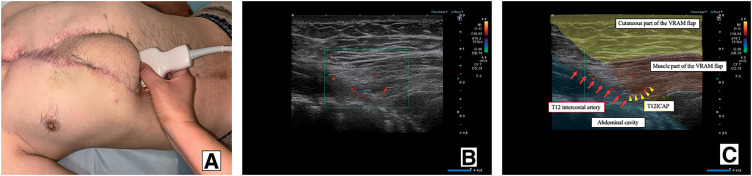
Fig. 4 (A) Postoperative color Doppler ultrasonography was performed to evaluate the flow of T12ICAP. (B, C) Postoperative assessment confirmed reliable perfusion through the T12ICAP to the transferred VRAM flap. T12ICAP: 12th intercostal artery perforator; VRAM: vertical rectus abdominis musculocutaneous
